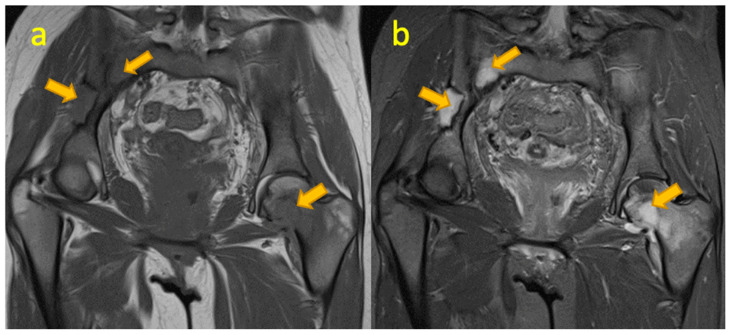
(a,b) Coronal T1W and STIR MR images of a 16-year-old female with left hip pain. There are multifocal osteolytic lesions in the left femoral neck, right iliac bone, and sacrum, later proved to be Langerhans cell histiocytosis.
